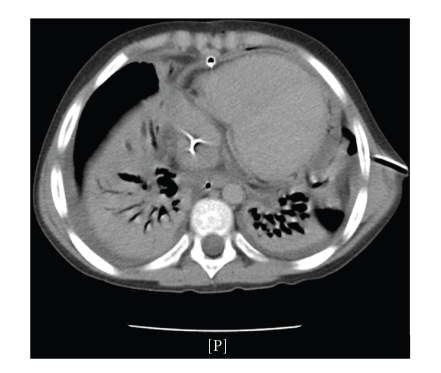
CT scan after subxifoid pericardial drainage.
